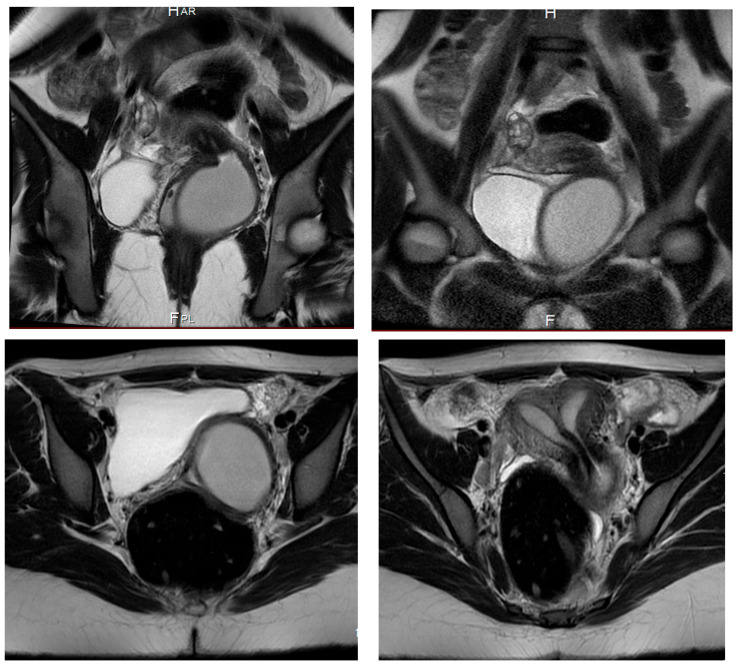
MRI pelvis (external study) in Case 1 demonstrating uterine didelphys with two cervices and a dilated left hemivagina filled with fluid due to distal vaginal obstruction. Absent left kidney is also noted.
